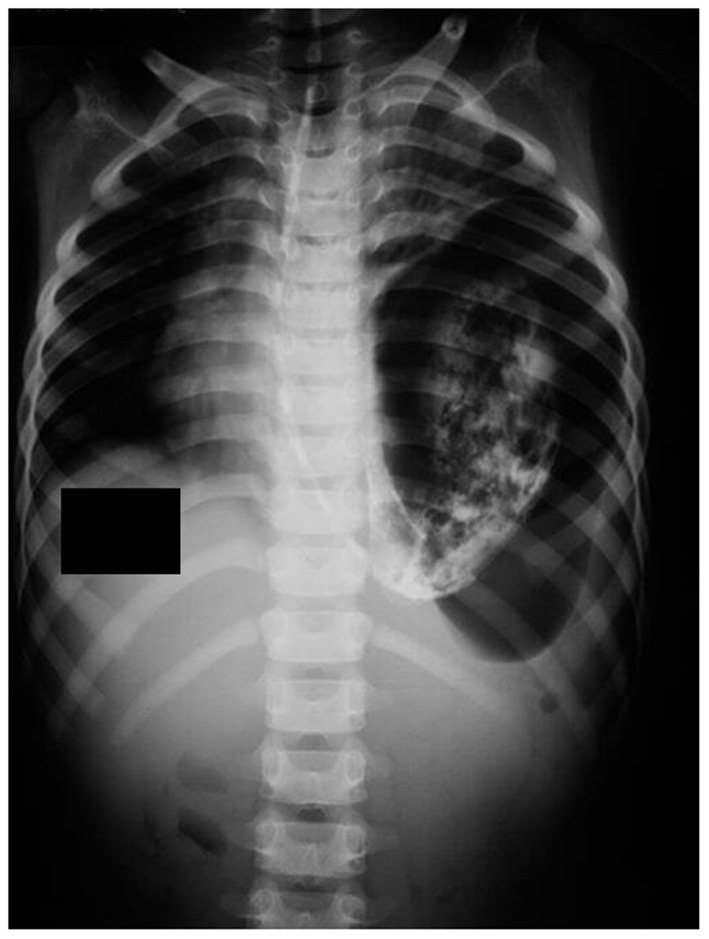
The barium-filled chest x-ray shows the NG tube and the contrast present in the thoracic cavity, indicating a CDH.
